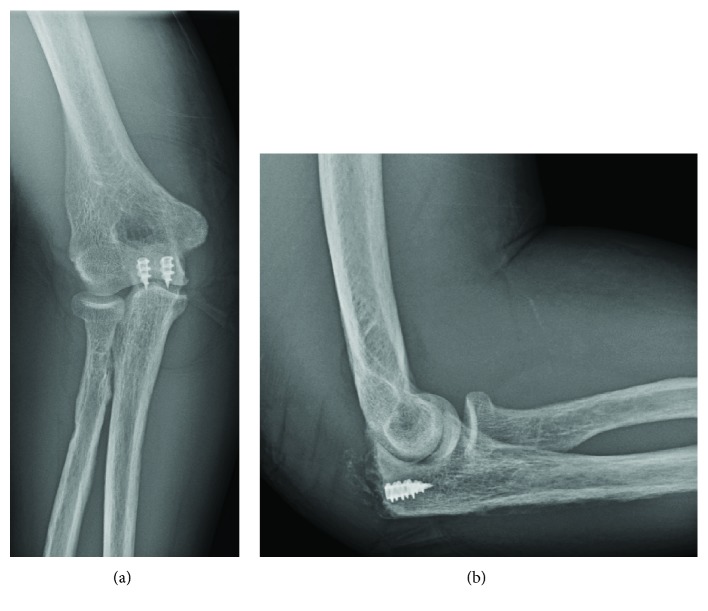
Postoperative (a) AP and (b) lateral X-ray of the elbow joint, showing 2 anchors in the olecranon, where sutures are anchored.
